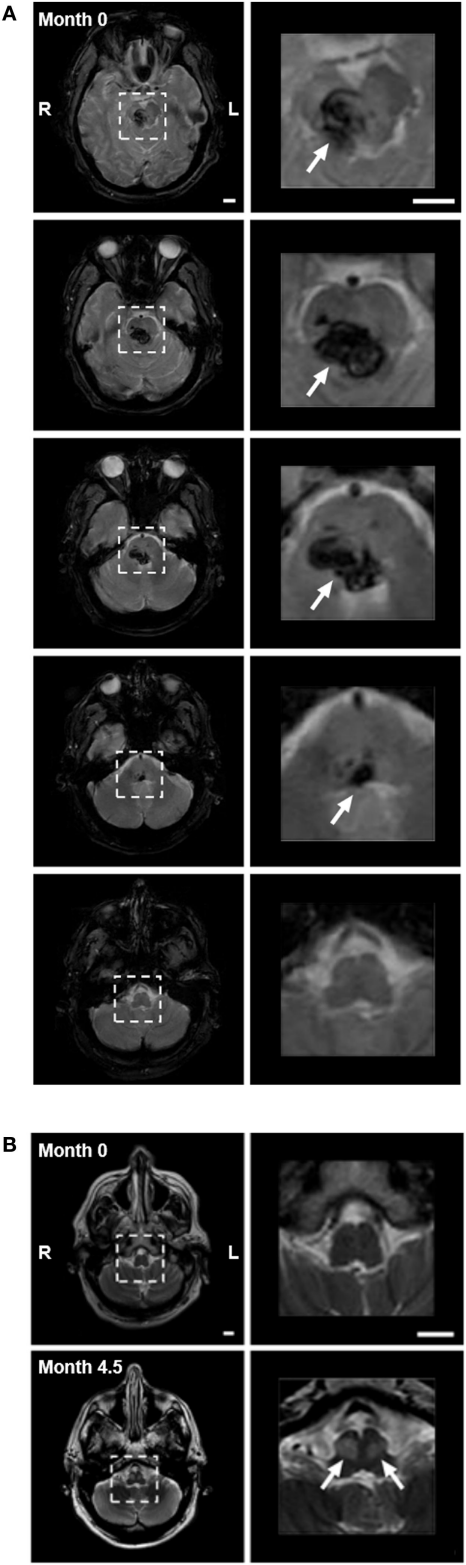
(A) Pontine hemorrhage imaged with gradient echo T2-weighted magnetic resonance imaging (MRI) within 24 h of hemorrhage. Left panels show consecutive 5-mm axial slices through the brainstem. Dashed squares highlight the region of hemorrhage. Right panels show 3.5× magnification of the highlighted region, with arrows indicating the hemorrhage (dark). The hemorrhage involved the pons and midbrain but not the medulla, primarily on the right side. (B) Axial images with turbo-spin echo T2-weighted MRI of medulla at time of hemorrhage (month 0—top panels), and after 4.5 months (month 4.5—bottom panels). Top panels: low (left) and higher (right) magnification images through the medulla (highlighted with dashed box) showed no evidence of hyperintensity at the time of hemorrhage. Bottom panels: bilateral focal hyperintensity of the anterior medulla at the level of the inferior olivary nucleus (arrows) was present at month 4.5, indicating the presence of hypertrophy. Scale bars = 1 cm.
